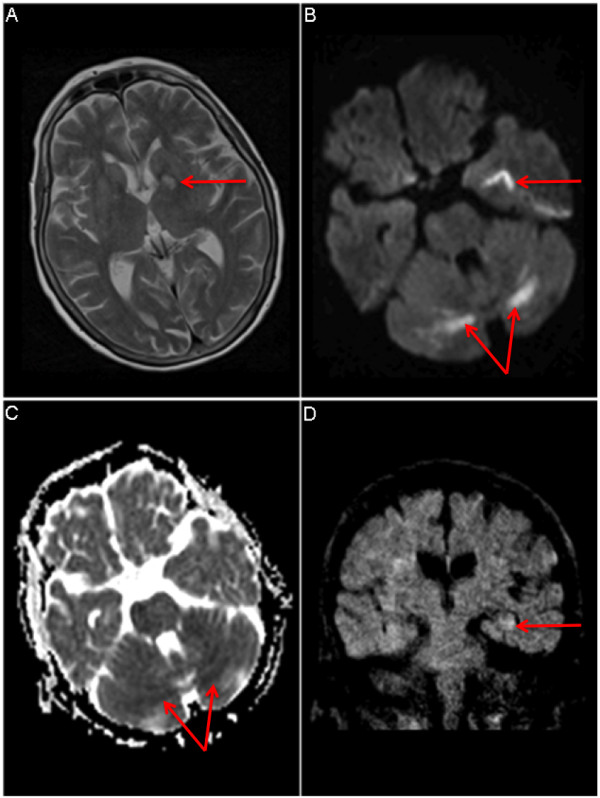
a T2-weighted MRI of brain with abnormal signal in the left medial temporal lobe (*arrow*). b Diffusion-weighted imaging (*DWI*) showing symmetrical bilateral restricted diffusion in cerebellar white matter (*arrow*) and in left medial temporal lobe (*arrow*). c Apparent diffusion co-efficient (*ADC*) map: corresponding areas are *dark* on ADC map, implying acute ischaemia. d Coronal reconstruction: Subtle signal abnormality in left hippocampus (*arrow*) possibly post-ictal in aetiology.
